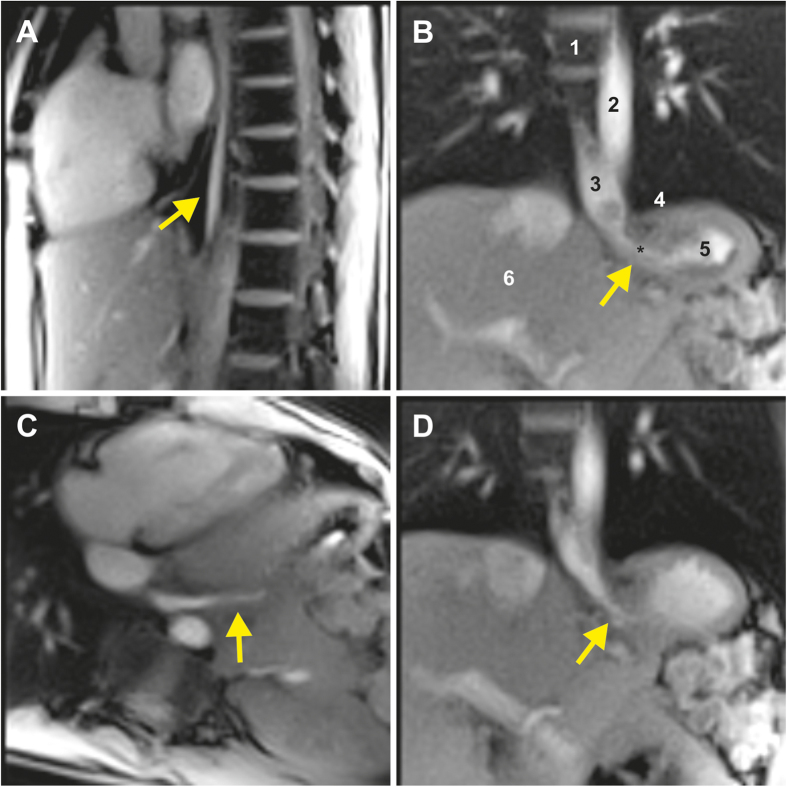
Real-time MRI of normal gastroesophageal swallowing in a 26-year-old healthy subject. Individual images (50 ms acquisition time, 2 × 2 mm^2^ resolution, 8 mm section thickness) were selected from corresponding movies (S3 to S6 in Supplementary Appendix) in (A) sagittal, (B) coronal oblique, (C) transversal oblique, and (D) coronal double-oblique planes along the directions of bolus transport (arrows). 1: spinal cord, 2: descending aorta, 3: lower oesophagus, 4: diaphragm, 5: stomach, 6: liver.
